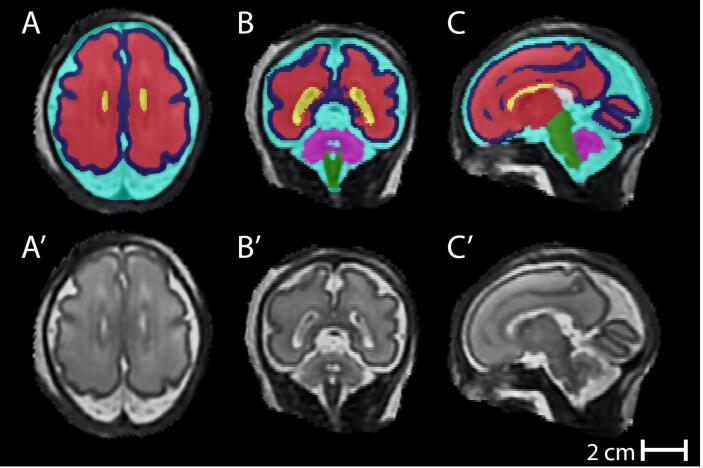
regional brain volumes in a control fetus at 28^+2^ weeks gestation acquired on a 3 T MRI system in the axial (A), coronal (B) and saggital planes (C) for supratentorial brain volume – red, lateral ventricles - yellow, extra-cerebral cerebrospinal fluid – turquoise, cerebellar volume - pink and cortical volume - blue. The original reconstructed datasets can be seen in A’, B’ and C’. (For interpretation of the references to colour in this figure legend, the reader is referred to the web version of this article.)
